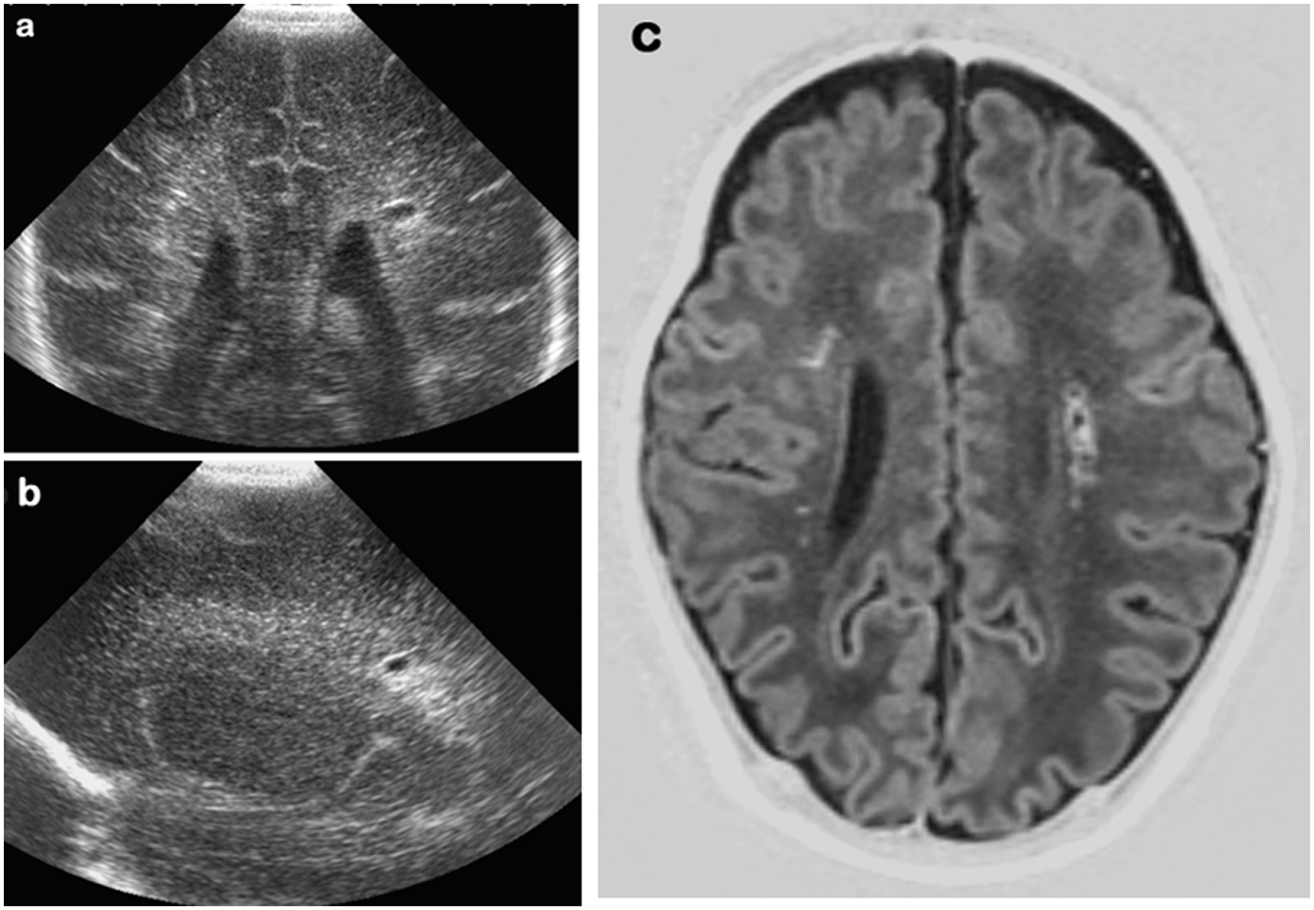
28 week gestation infant; cUS at 5 weeks and TEA-MRI. cUS, coronal (a) and left parasagittal (b) views showing a single cyst and echogenicity surrounding the cyst. T1-weighted MRI at TEA confirming the cyst and several focal lesions of increased signal intensity bilaterally (c).
